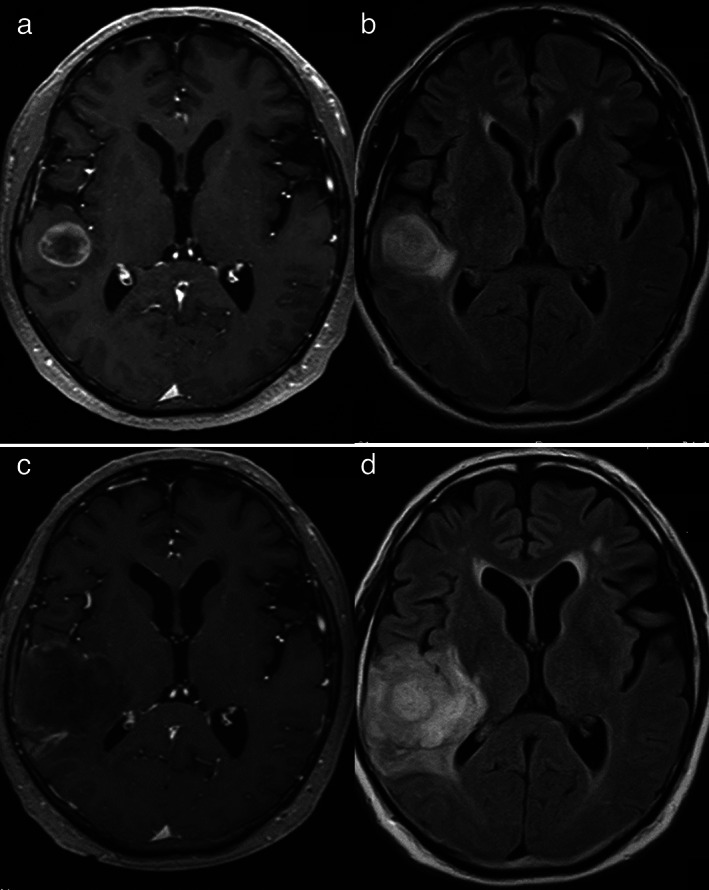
Brain magnetic resonance imaging nine and 14 months after pneumonectomy. (a) A brain metastasis in the right temporal lobe nine months after pneumonectomy (T1‐weighted image (WI) with gadolinium (Gd) enhancement). (b) Brain edema around the tumor nine months after pneumonectomy (fluid‐attenuated inversion recovery [FLAIR]). (c) Increase in brain tumor size 14 months after pneumonectomy (T1‐WI with Gd enhancement). (d) Worsening perifocal edema 14 months after pneumonectomy (FLAIR)
